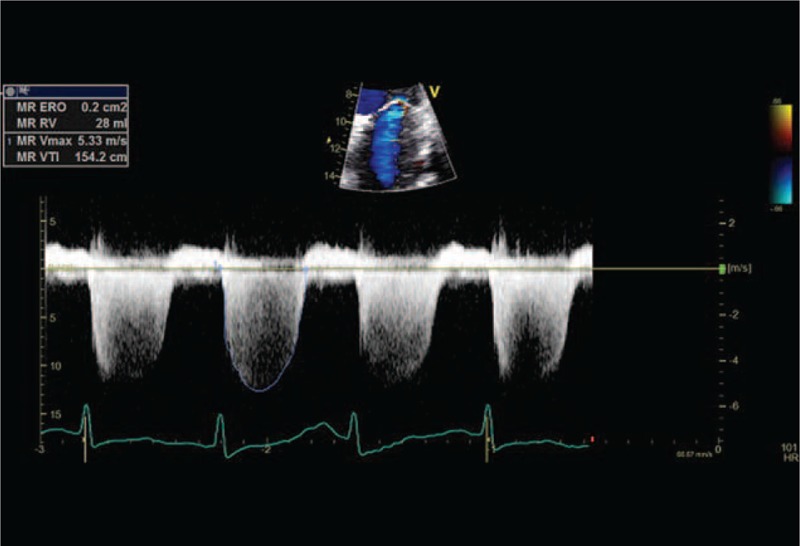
MR during cycle-stress-echocardiography (EROA = 0.2 cm^2^, RV 28 mL). EROA = effective regurgitant orifice area, MR = mitral regurgitation, RV = regurgitant volume.
